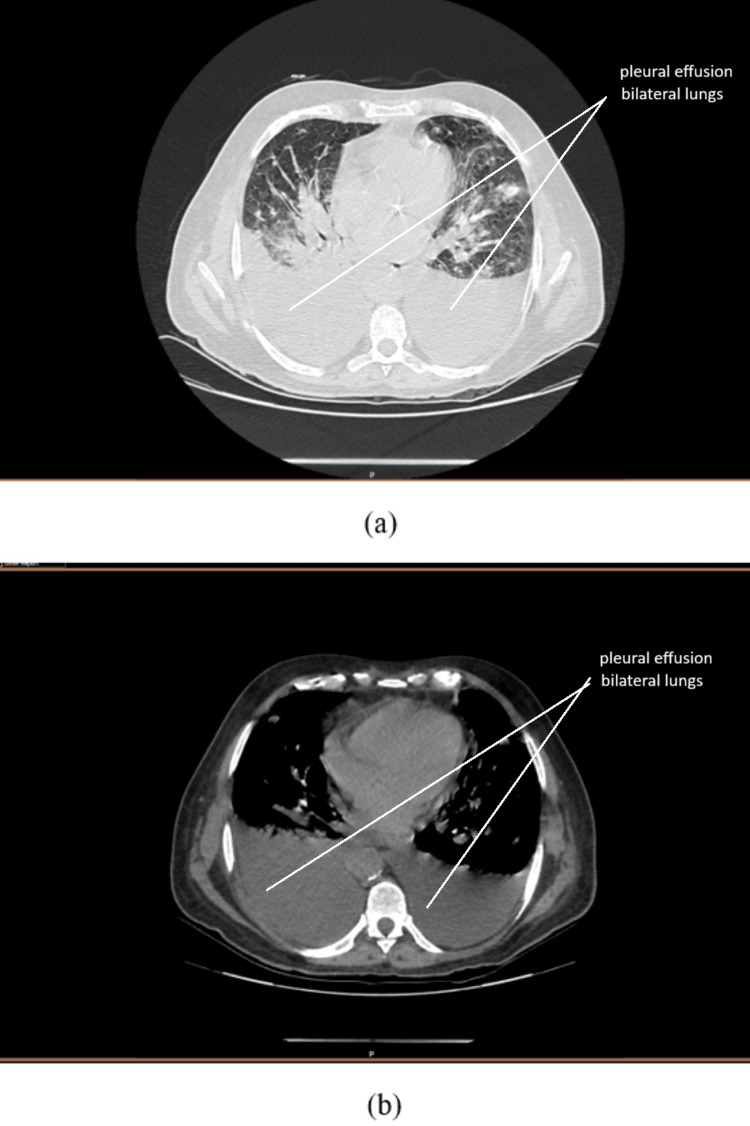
CECT thorax CT revealed a large right-sided effusion and a moderate left-sided effusion, with partial collapse of the bilateral lower lobes. The visualized lung fields showed multiple metastatic nodules of varying sizes. Few patches of consolidation were seen centrally in the lung fields, likely of infective etiology. An enlarged left kidney with perirenal fat stranding and thickened Gerota's fascia, consistent with the known neoplastic status, was also observed. CT, computed tomography; CECT, contrast-enhanced computed tomography
